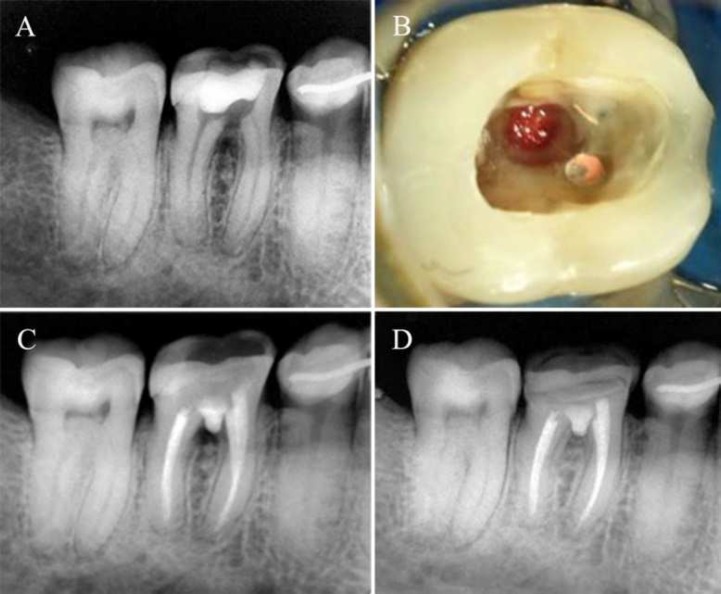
A)* Initial radiographic image; *B)* Furcal perforation clinical image; *C)* Immediate radiographic image after perforation sealing; *D)* Radiographic image after 180 days follow-up
